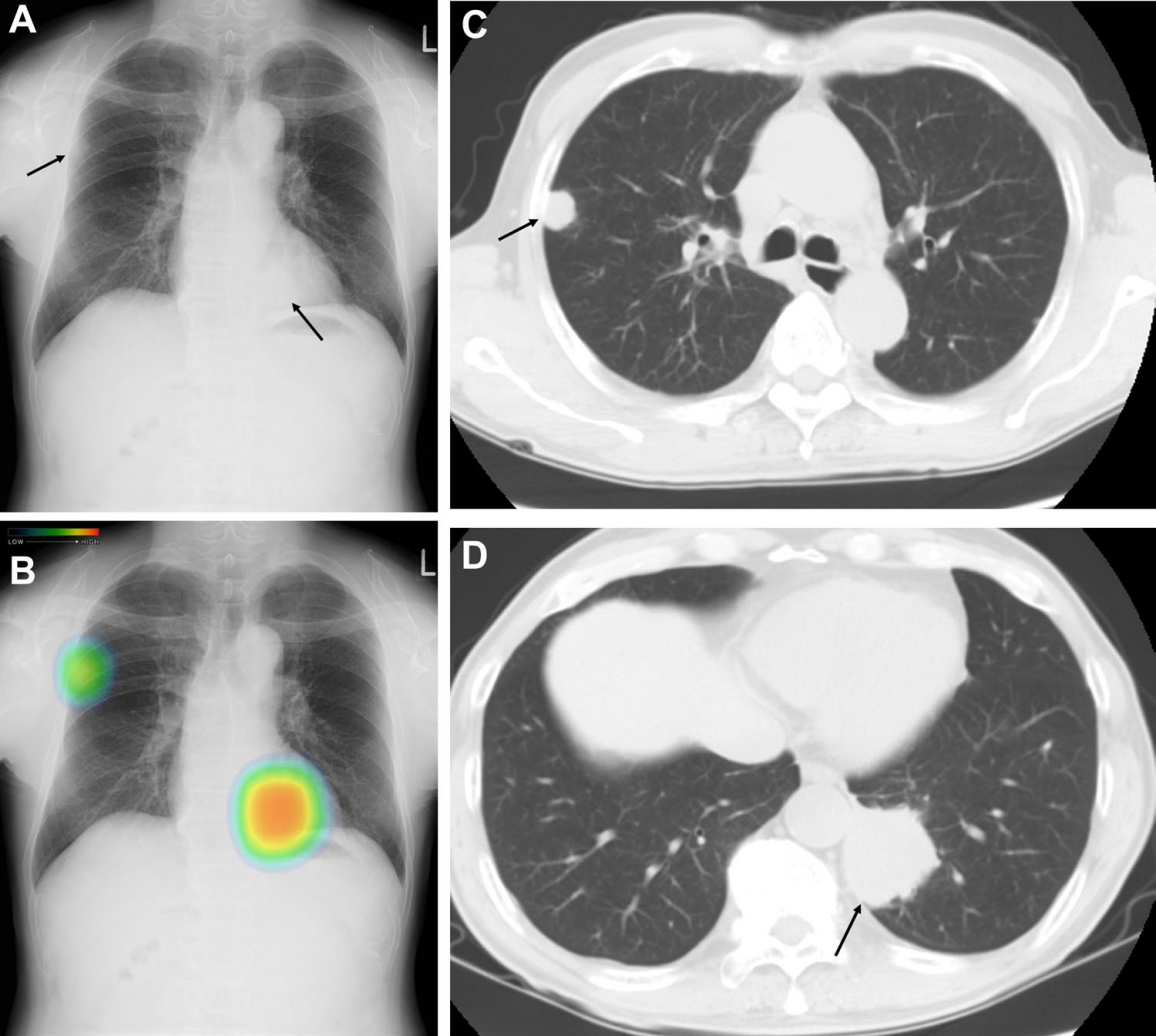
Nodule detection on chest radiograph by a commercially available AI-based CAD system. A 70-year-old man. A nodule can be seen in the right middle lung field and a mass can be seen in the left lower lung field overlapping the cardiac shadow (arrows, A). A commercially available AI-based CAD system can highlight both the nodule and mass. A nodule with 2 cm in diameter can be seen in the right upper lobe on CT (arrow, C). A mass with 5 cm in diameter can be seen in the left lower lobe on CT (arrow, D). This mass was confirmed as a lung metastasis from renal cell carcinoma after biopsy. *AI* artificial intelligence, *CAD* computer-aided detection/diagnosis
